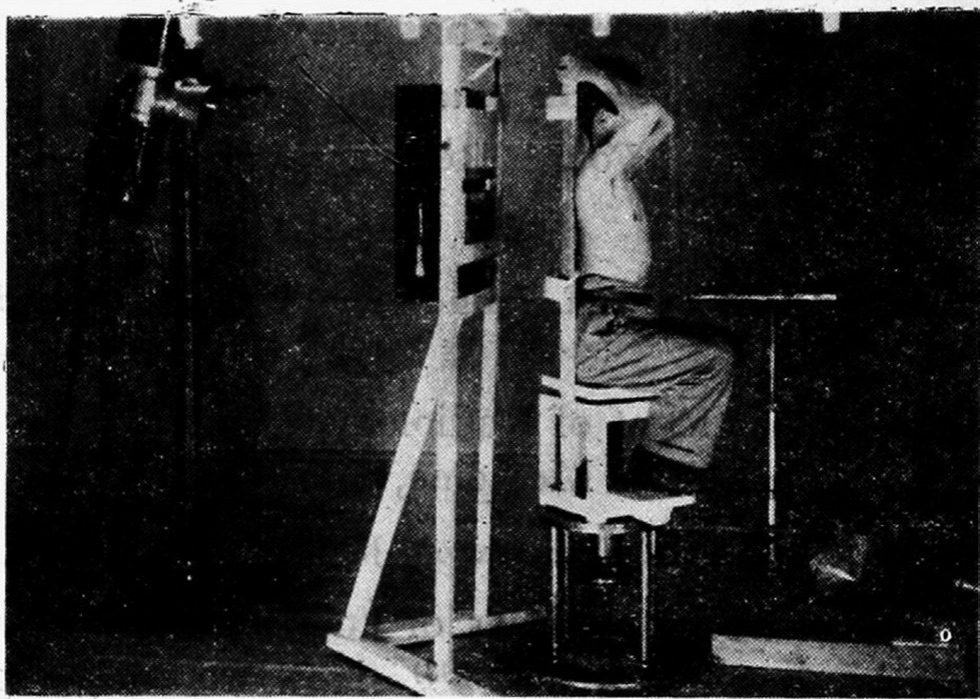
Rotational cross-section tomography. The film is placed on the turntable, and then the X-ray beam is used. *Note*. Reprinted from “Rotatory Crossgraphy”, by Takahashi S. et al, *The Tohoku J. of Experimental Medicine* 1951; 54:60, Figure 1
